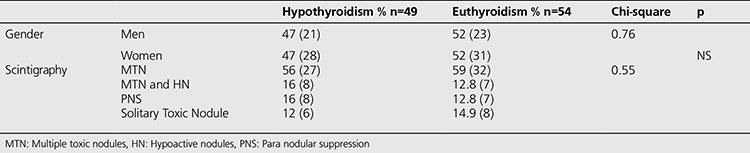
Effect of gender and scintigraphic pattern on outcome
